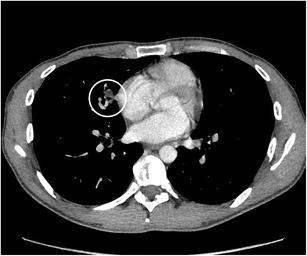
Axial CT-image in mediastinal window setting in a 33-year-old fire-eater shows a well delineated nodule in the right middle lobe with internal fat component. Findings are consistent with small lipoid pneumonia. The nodule completely resolved after treatment
